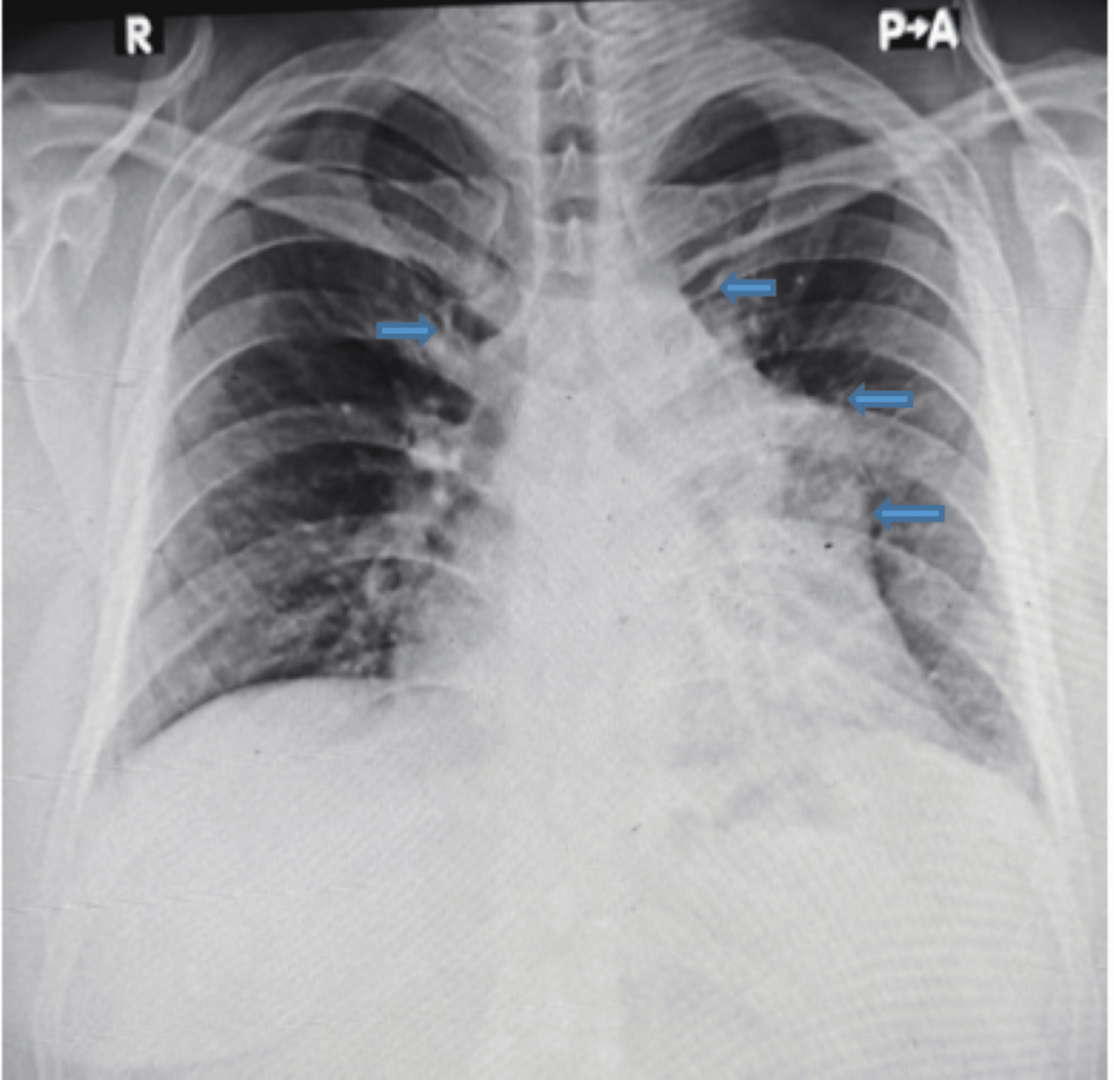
Chest radiograph (PA view) at presentation showing mediastinal widening PA, posteroanterior
